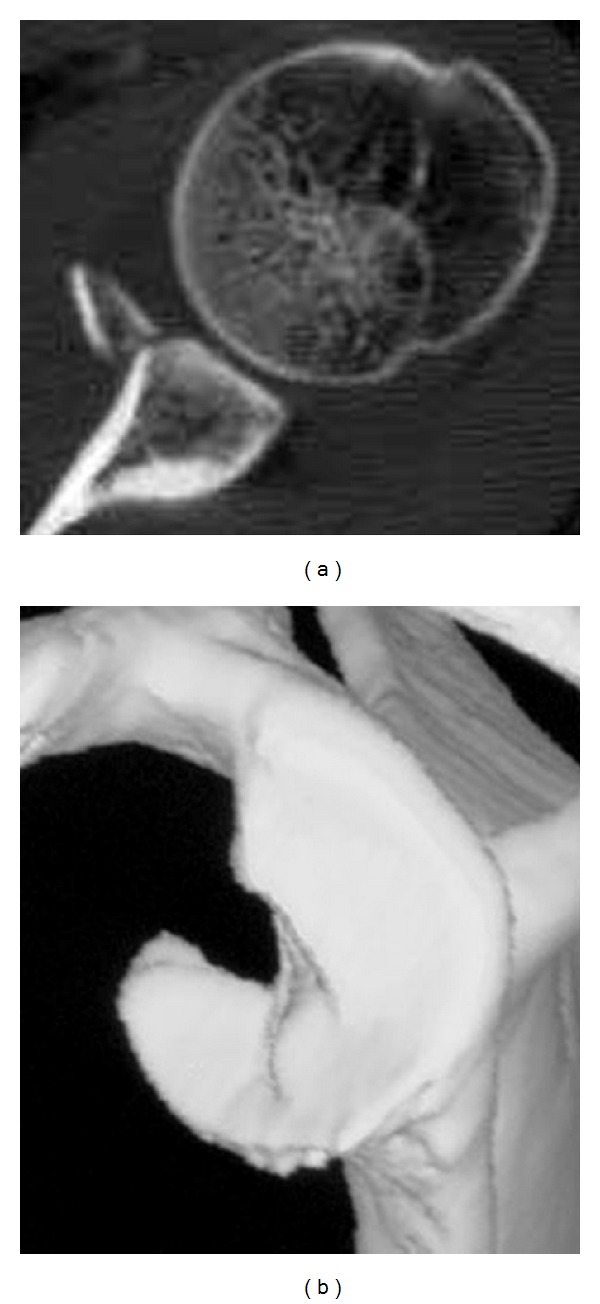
CT imagingat the time of injury. Axial image of CT shows a bony Bankart lesion at the anteroinferior part of glenoid articular surface (a). Size of the bony fragment was 17 mm × 27 mm and its width was 41% of the glenoid length on the 3-dimensional CT (b).
